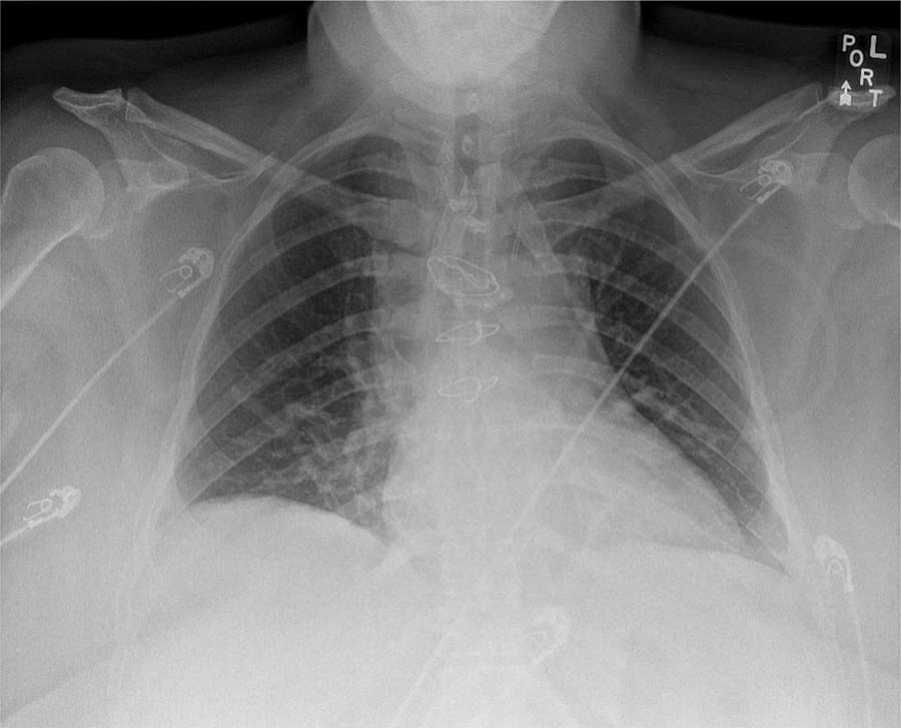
Postoperative chest X-ray demonstrating sternal wire and sternal plate closure.
